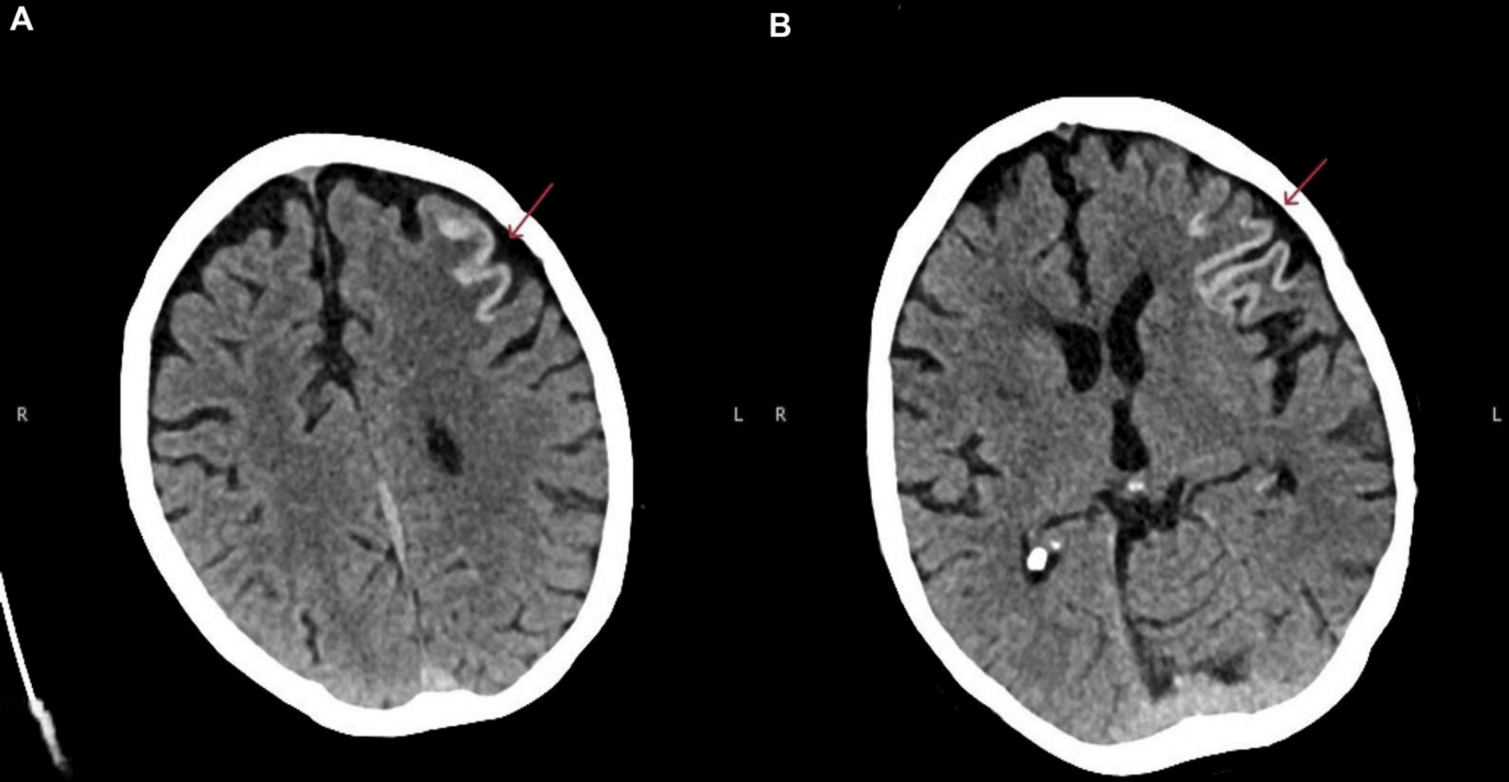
Axial non-contrast computed tomography (CT) of the head showing cortical laminar necrosis in the left frontal lobe, with hyperattenuation along the cortical ribbon consistent with irreversible ischemic neuronal injury.
